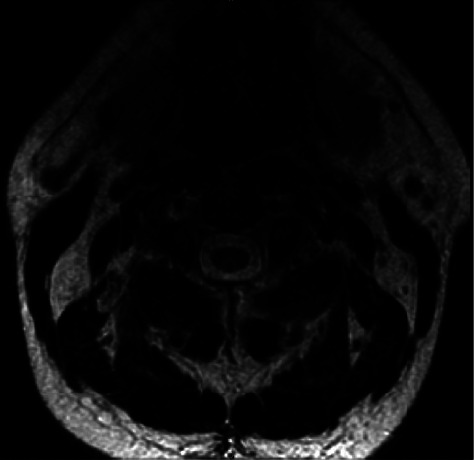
MRI of the cervical spine showing abnormal signal in the posterior columns.
